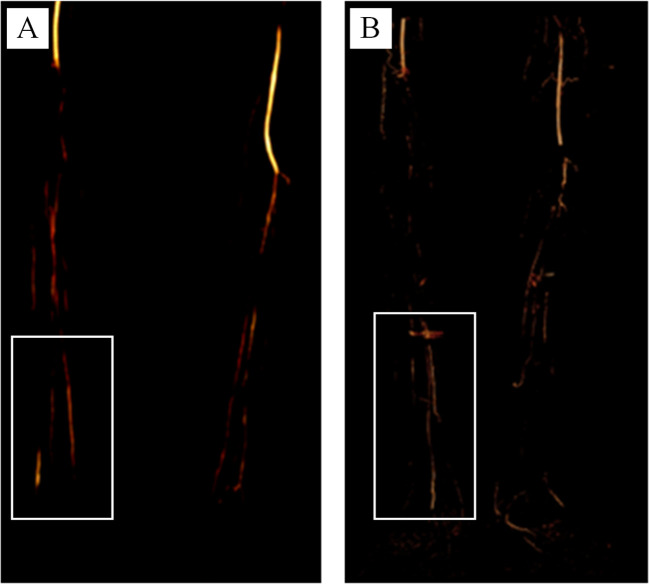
Three-dimensional CTA reconstruction in the same 46-year-old male patient with Wagner grade IV DFU (see Figure 8). (A) Preoperative CTA. (B) CTA at 2 months postoperatively.
